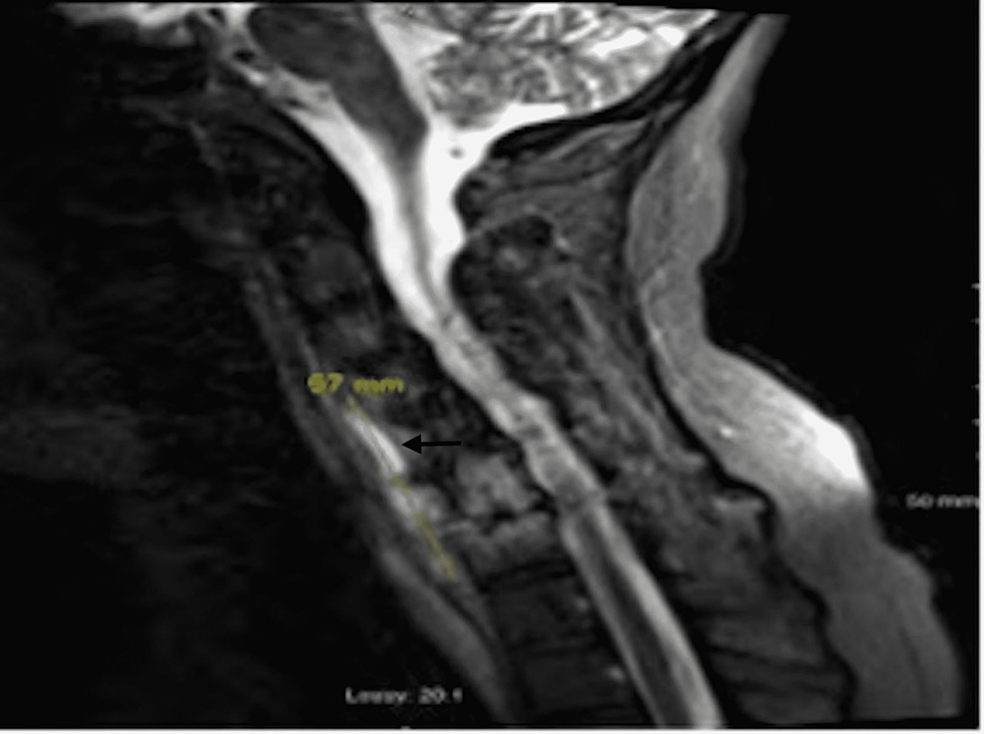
MRI Cervical Spine Image showing area of abnormal signal within the disc space and marrow at C5-C6 and C6-C7, marked by an arrow, concerning for discitis-osteomyelitis.
